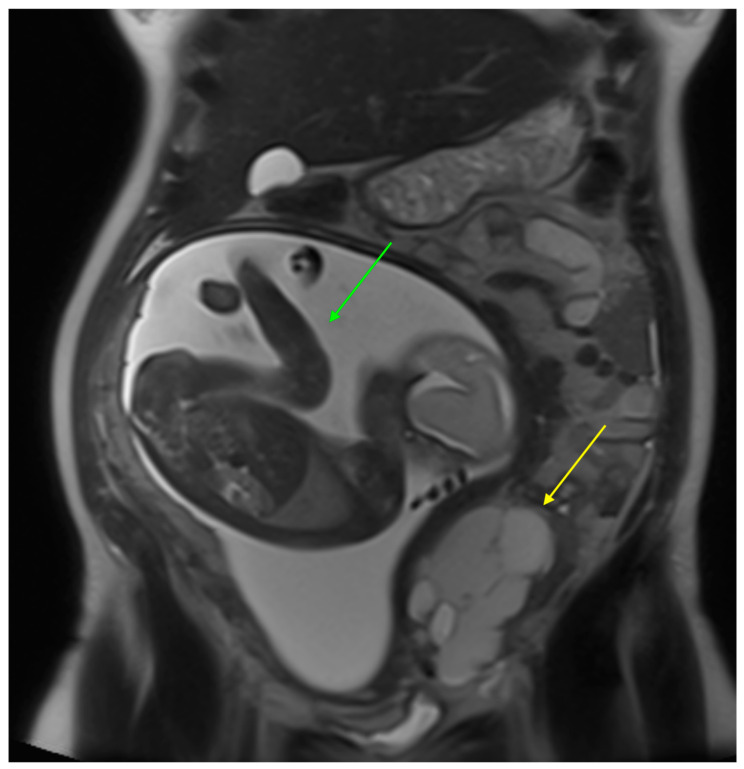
Abdominal MRI, sagittal view of the fetus (green arrow) and a high-grade serous ovarian cancer (yellow arrow).
